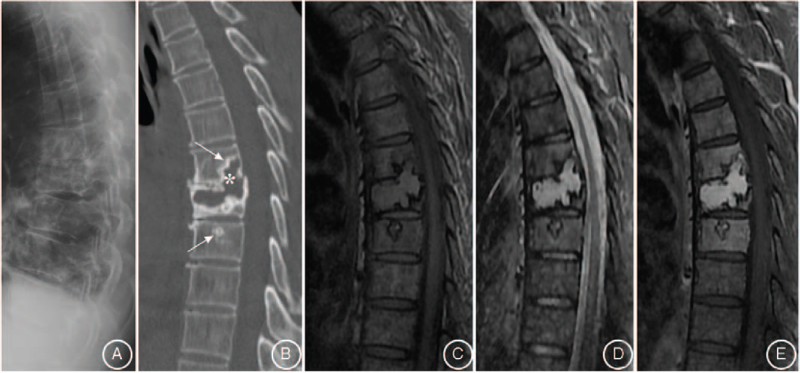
Lateral spine radiograph demonstrated osteolytic destruction of T7 and T8 vertebral body with intervertebral space stenosis (A). Mid-sagittal CT of thoracic spine suggested slight expansile osteolytic destruction in T8 vertebral body with sclerosis margin, extension to T7 and T9 vertebral body (arrows) and adjacent disk-space destruction (asterisk) (B). The corresponding thoracic spine MRI revealed the lesion exhibiting mildly isointense on T1 weighted image (C), hyperintense on T2 weighted image (D), and intense homogeneous enhancement on T1-weighted post contrast image (E).
